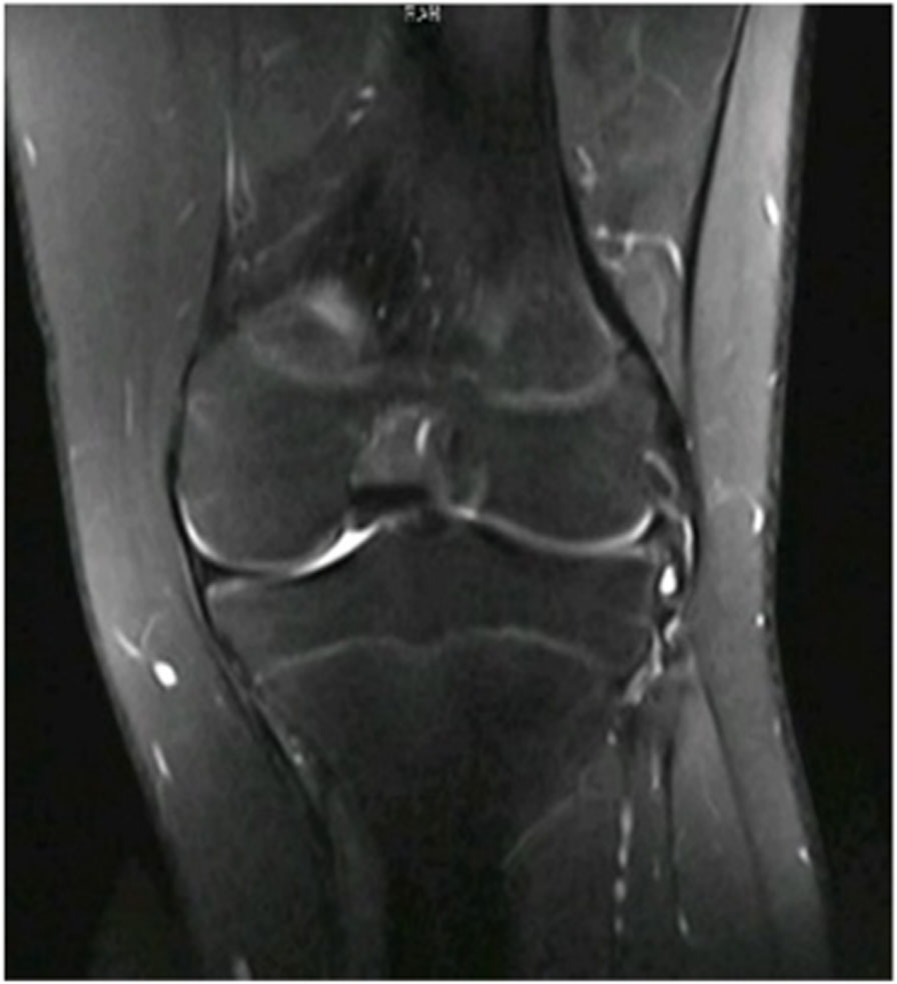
Absence of high signal intensity at the remnant menisci in T2-weighted MRI
